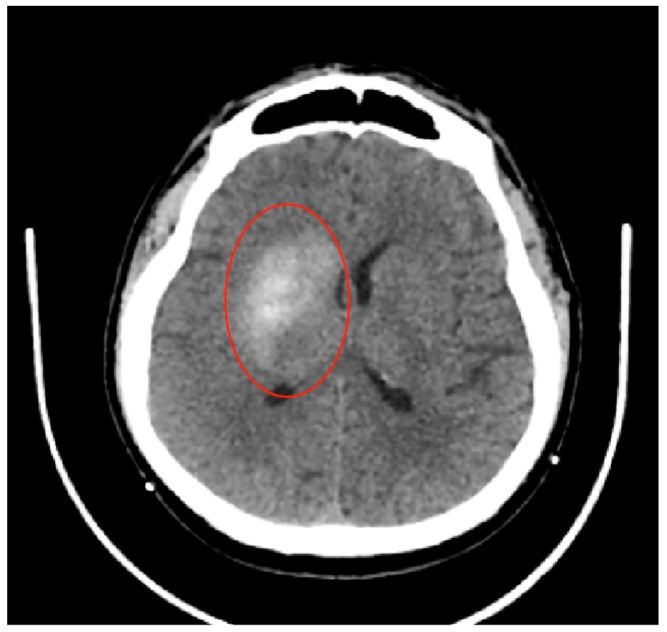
Head CT scan performed at 24 h after mechanical thrombectomy showed residual contrast substance in the nucleus basalis after revascularization—without established cerebral ischemia (red circle). The PC-ASPECTS was 10.
